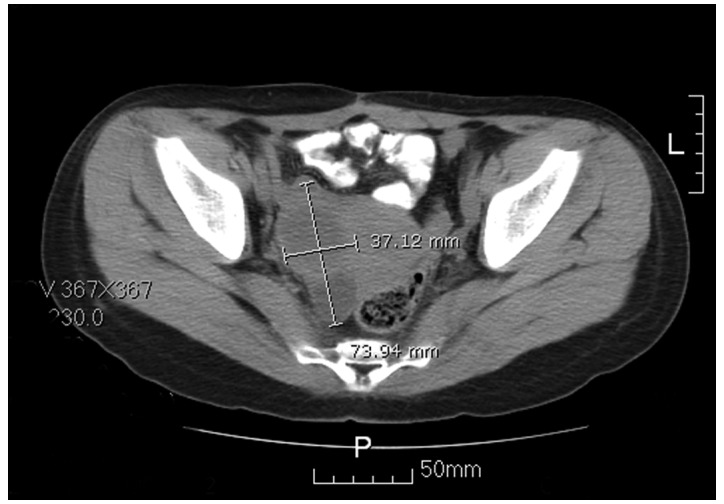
Computed tomography revealed the cystic and solid mass in the right parametrium markedly increased (measuring 3.7×7.3 cm).
